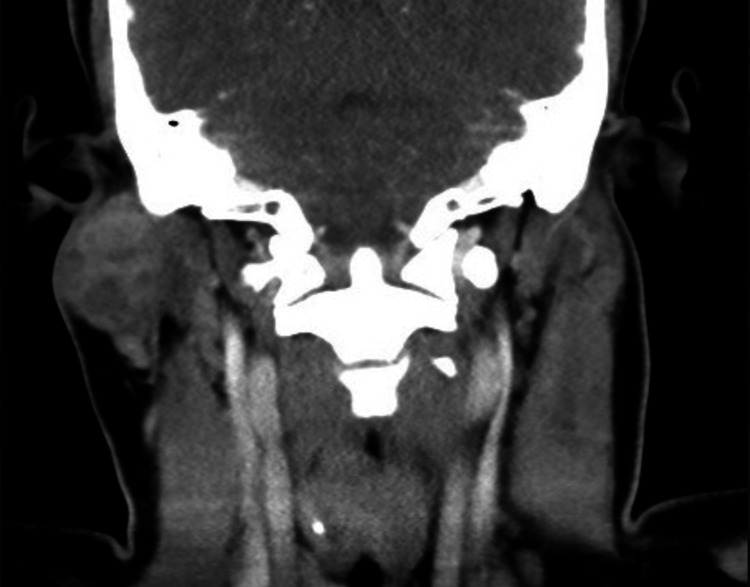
Contrast-enhanced computed tomography (axial image) After administering the contrast medium, a slight enhancement of the lesion was identified, better delimiting the areas with cystic degeneration.
